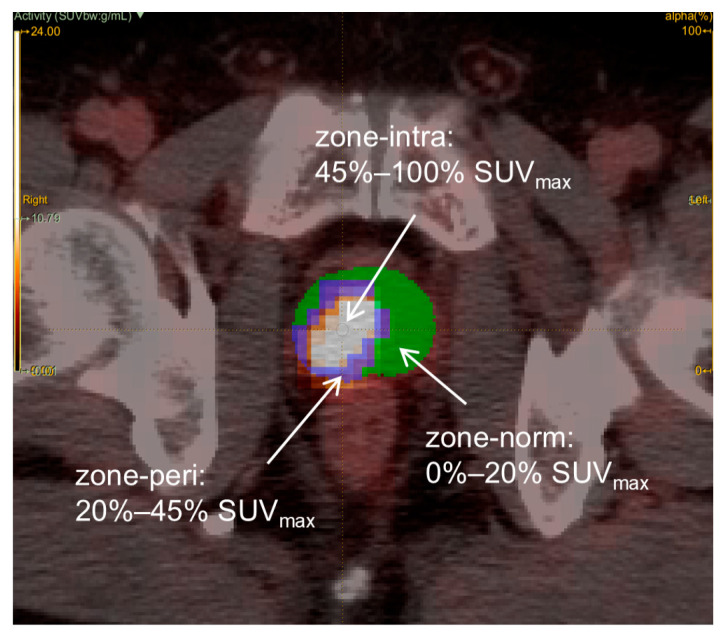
The different subregions delineated of the prostate on ^18^F-PSMA-1007 PET/CT.
